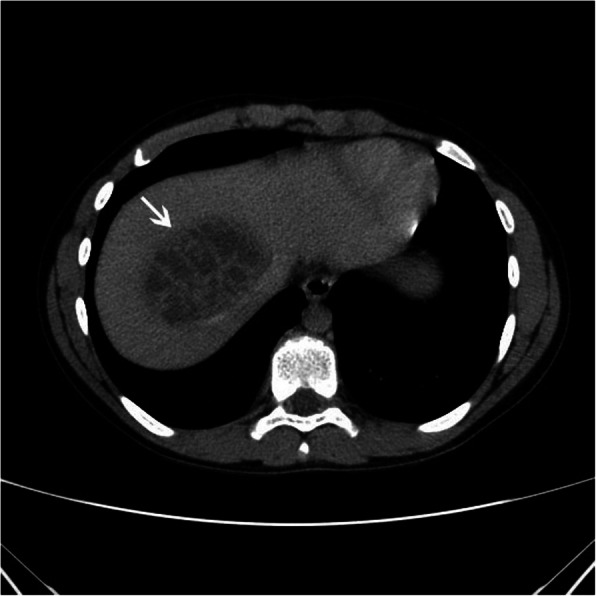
Axial contrast-enhanced portal phase CT image shows a single, low-attenuation, multilocular lesion in the segment VIII of the liver
